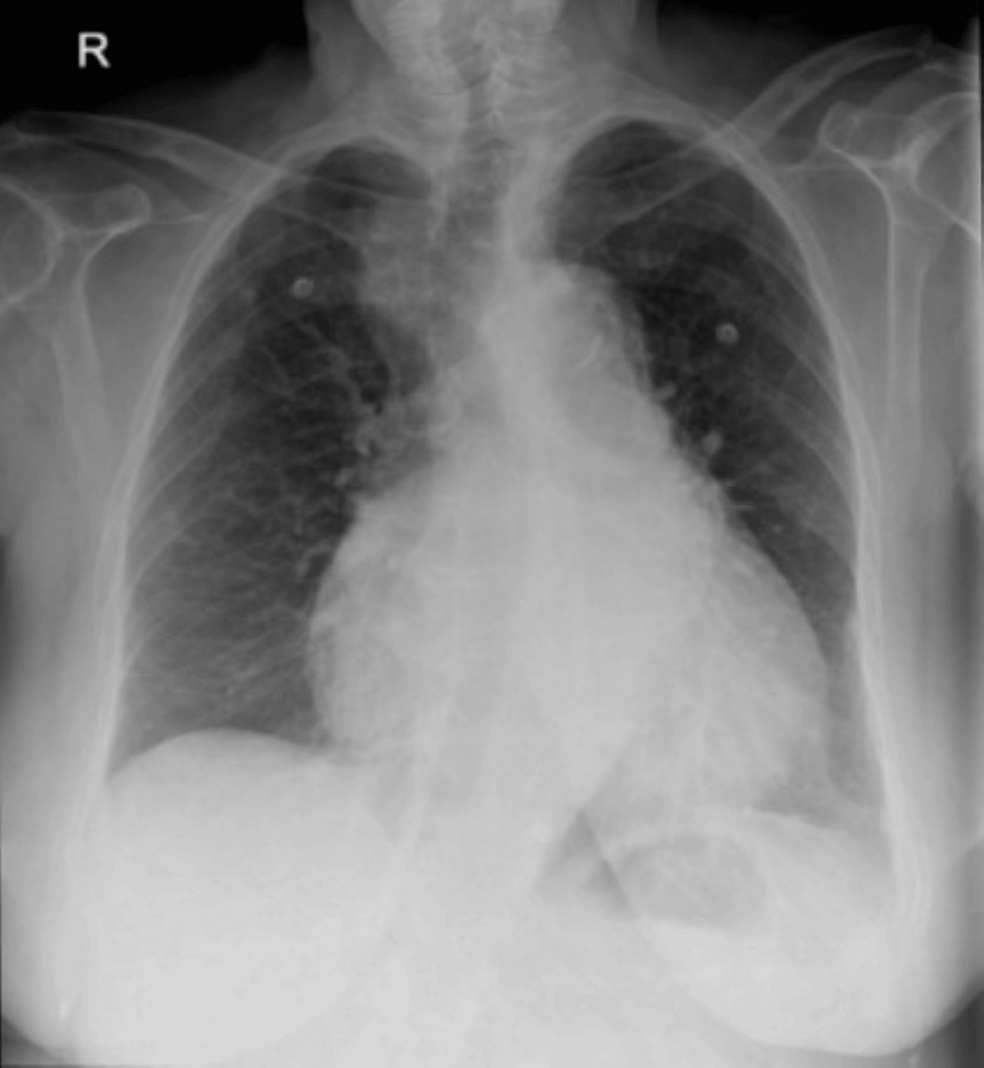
Chest X-ray Chest X-ray reveals normal mediastinum width and cardiomegaly; the patient was known to have cardiomegaly.
